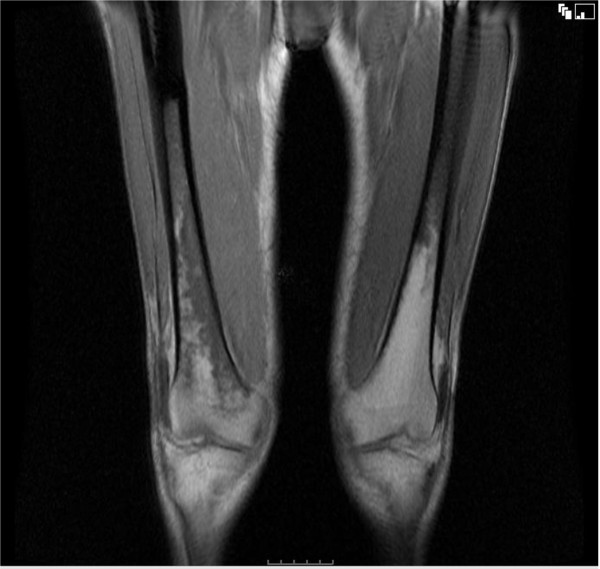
MRI scan showed an increased marrow signal in the distal femur and proximal tibia.
